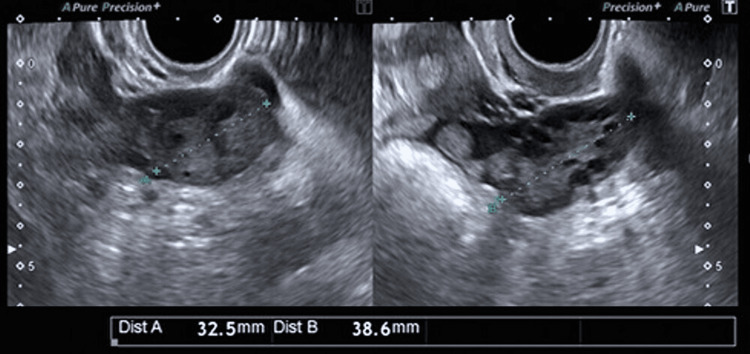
Follow-up transvaginal ultrasonography one month after surgery. The right ovary (left image) has decreased in size to 32.5 mm and returned to normal size.
